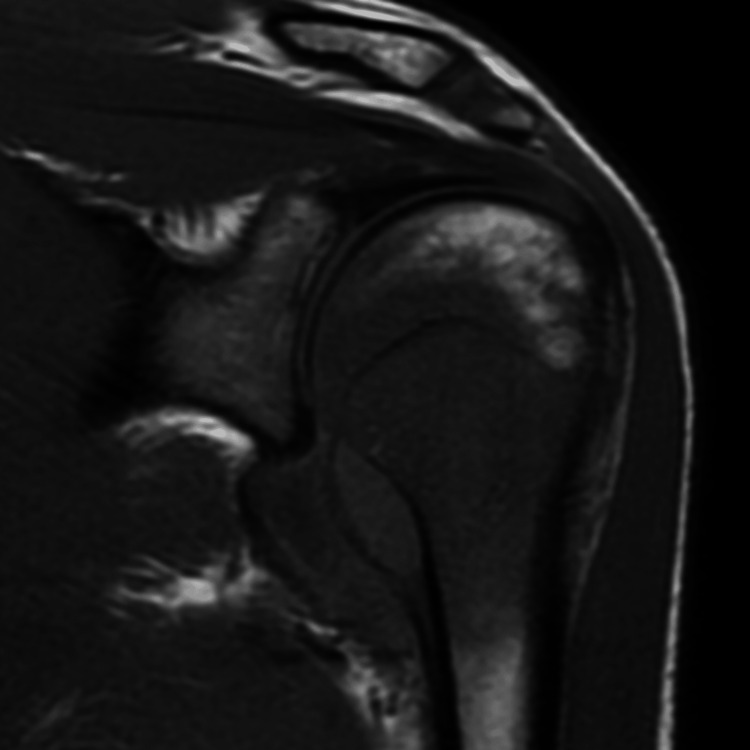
T1-weighted coronal image of the left shoulder A large mass is seen in the head and neck of the humerus with subperiosteal component. Also seen is elevated periosteum.
